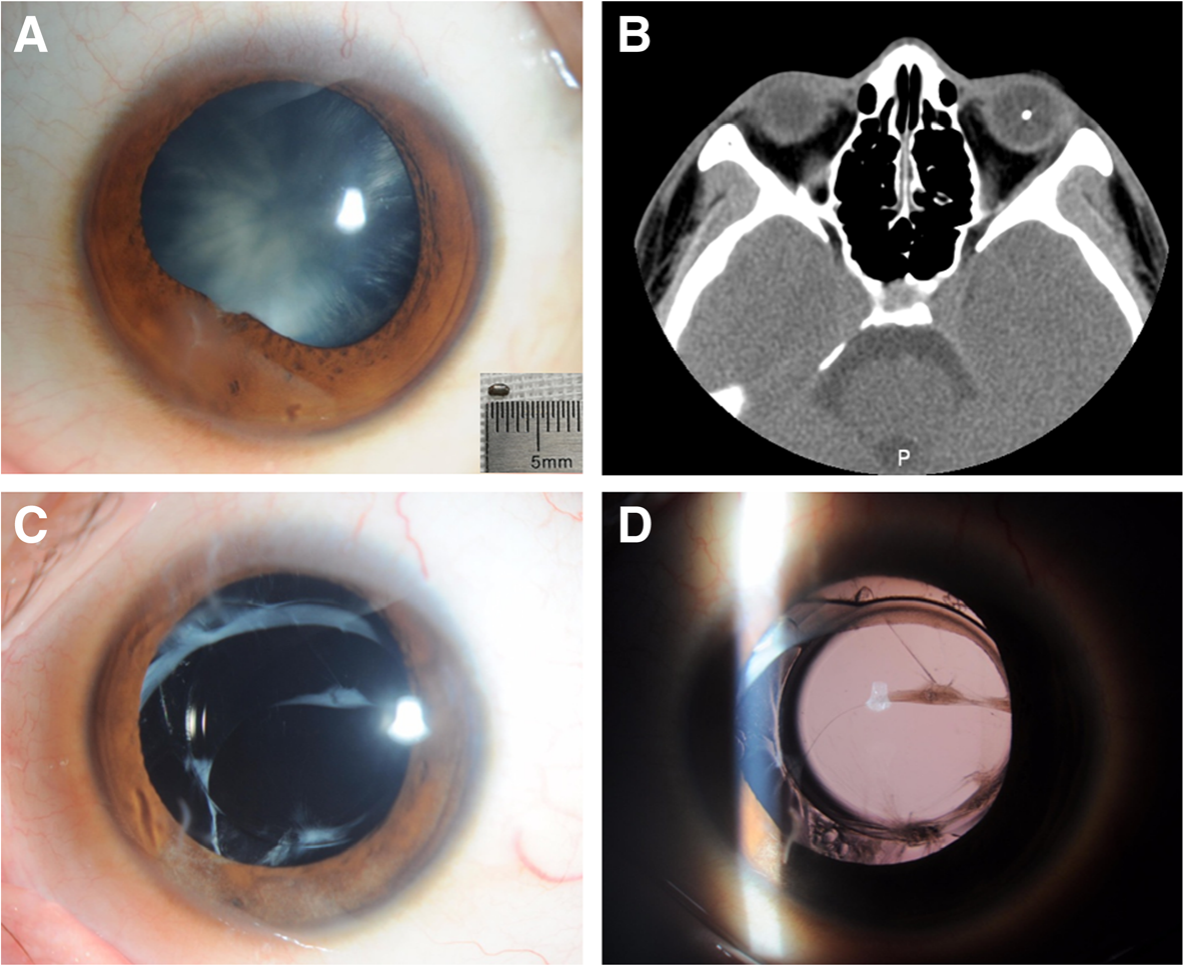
Patient 9 with total cataract. a: Anterior segment photograph revealed a peripheral self-sealing corneal penetrating wound at 7 o’clock position, iris posterior synechia, and total cataract. The size of foreign body was 1.5 mm in width and 2.0 mm in length. b: A small metallic-like foreign body, suspended in the vitreous cavity, was identified by orbital computed tomography. c, d: The IOL was well centered 12 months after secondary removal of traumatic cataract and implantation of IOL
